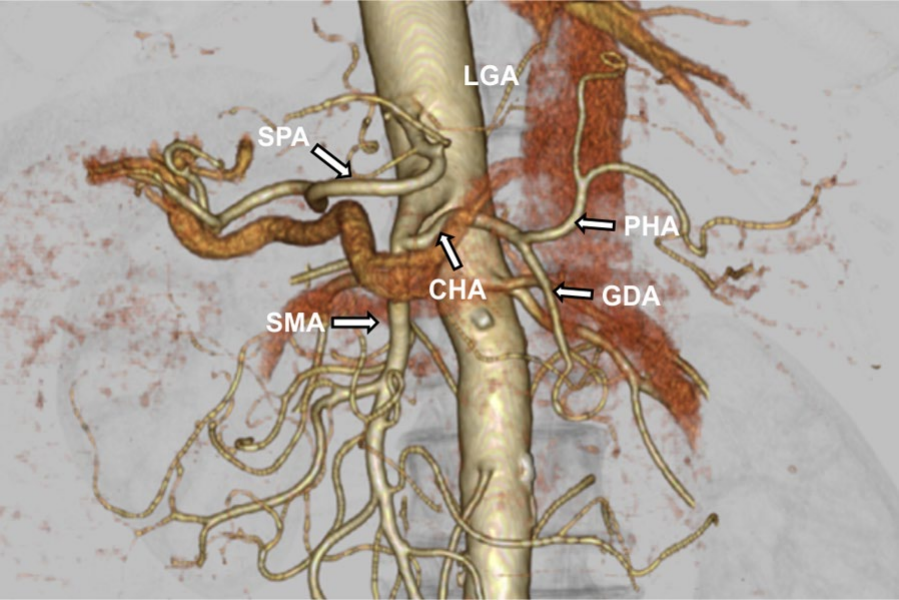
Three-dimensional reconstruction image of CT angiography preoperative findings. The common hepatic artery (CHA) originated from the SMA. *CHA* common hepatic artery, *SPA* splenic artery, *SMA* superior mesenteric artery, *LGA* left gastric artery, *PHA* proper hepatic artery, *GDA* gastroduodenal artery
